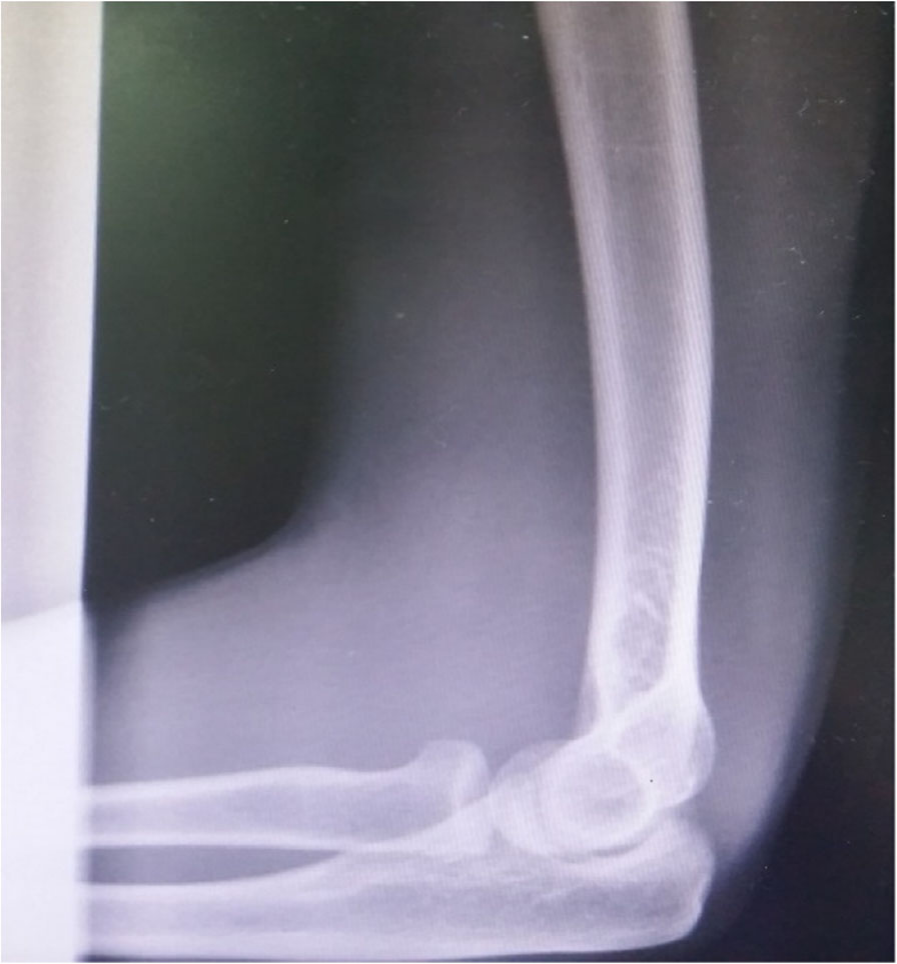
Lateral view radiograph of left elbow
